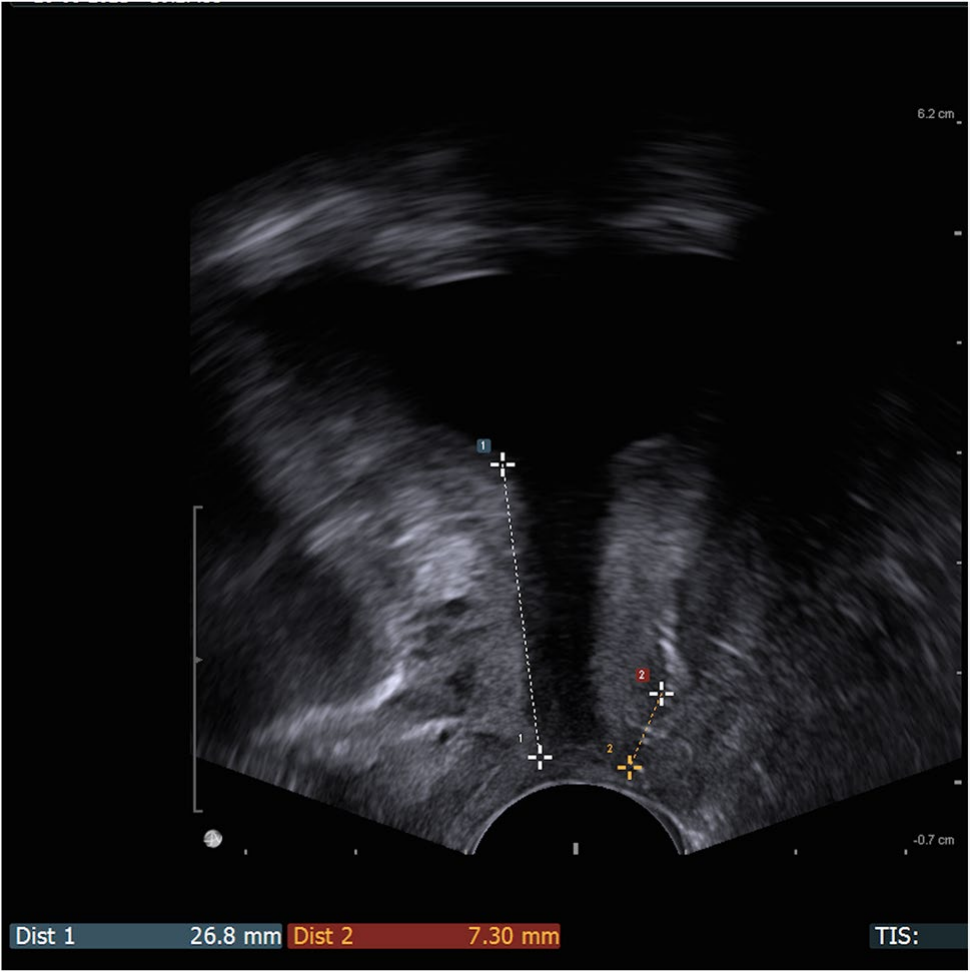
Ultrasonographic tapevisualisation in mid-sagittal scan: Dist 1: urethral length (U), Dist 2: distance from the external urethral orifice to the lower edge of the tape (T)
